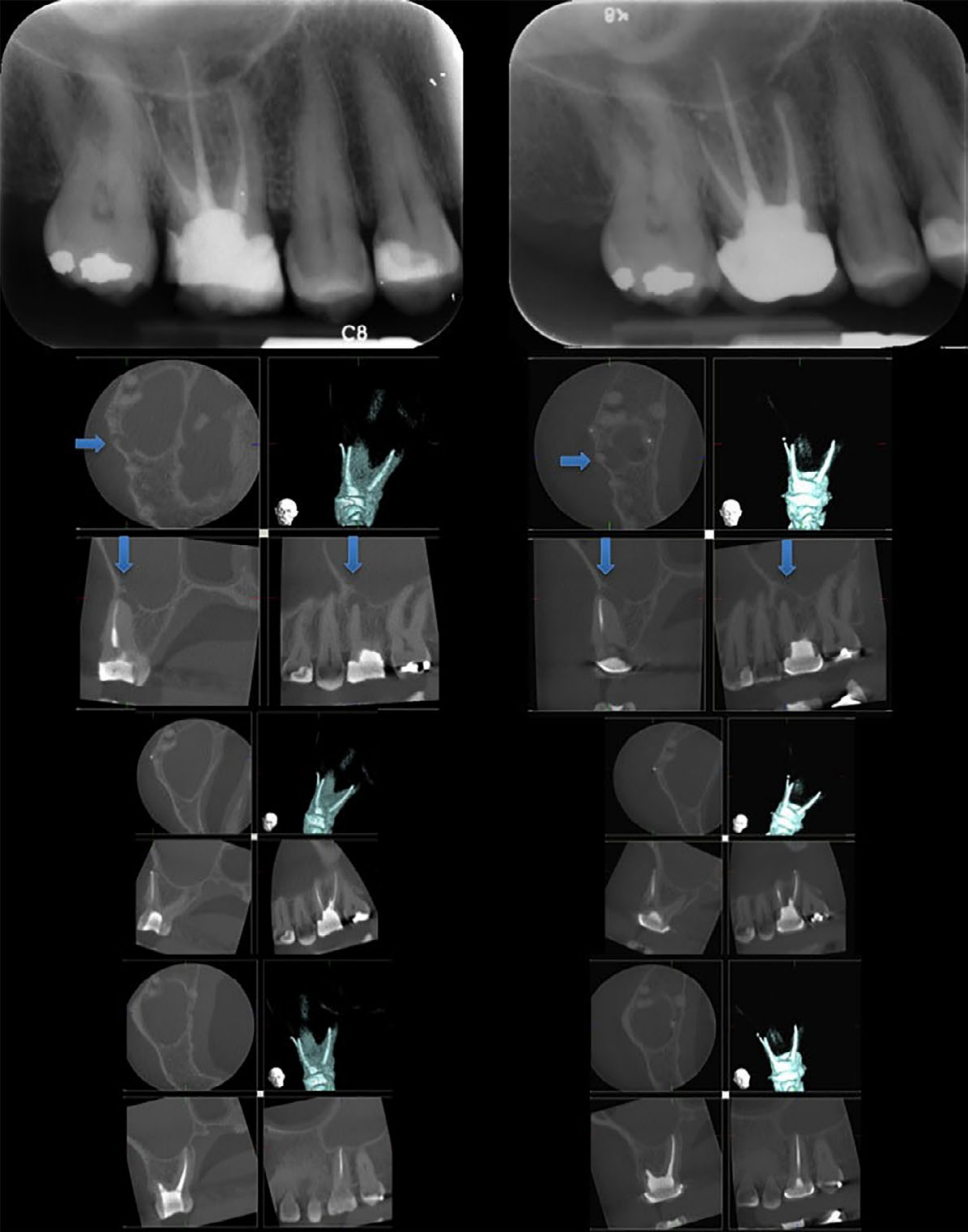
Item 11g – Example of a case detailing all the images made available for the examiners. They had the entire CBCT scan to view if they wished. (a, b) Preoperative and 1 year postoperative periapicals of the 16 showed apparent reduction of the periapical lesion associated with the mesial root. (c, d) Preoperative and 1 year postoperative reconstructed images of the 16 mesial roots in axial, coronal and sagittal planes showing enlargement of the periapical lesion associated with the root (arrowed). (e,f) Preoperative and 1 year postoperative reconstructed images of the distal root showing maintenance of a healthy periapex. (g, h) Preoperative and 1 year postoperative reconstructed images of the palatal root showing maintenance of a healthy periapex. Reprinted from *International Endodontic Journal*, Vol 49, Davies, A., Patel, S., Foschi, F., Andiappan, M., Mitchell, P. J., & Mannocci, F. (2016). The detection of periapical pathoses using digital periapical radiography and cone beam computed tomography in endodontically retreated teeth – part 2: A 1‐year post‐treatment follow‐up, pages No. 623–635, Copyright (2016) with permission from Wiley.
